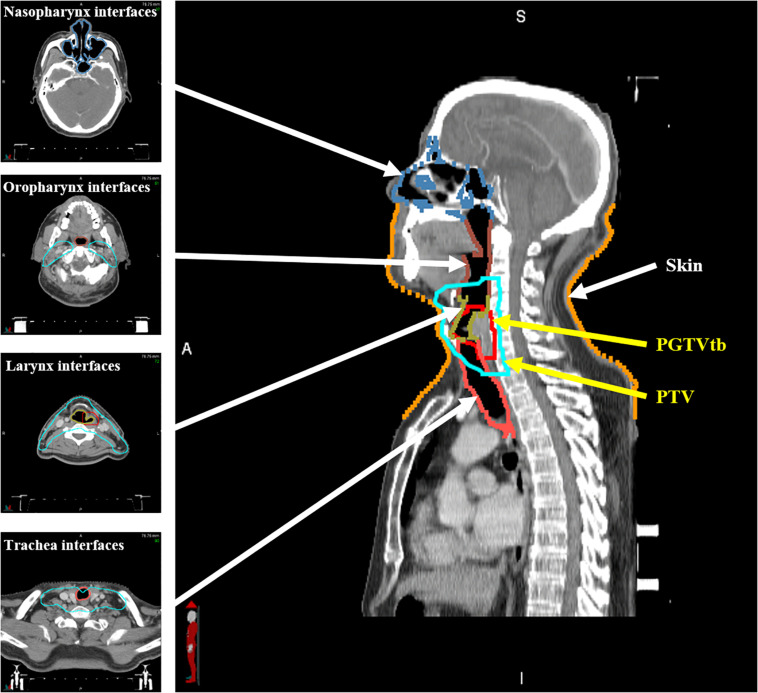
A patient CT scan with delineated regions of interest.
